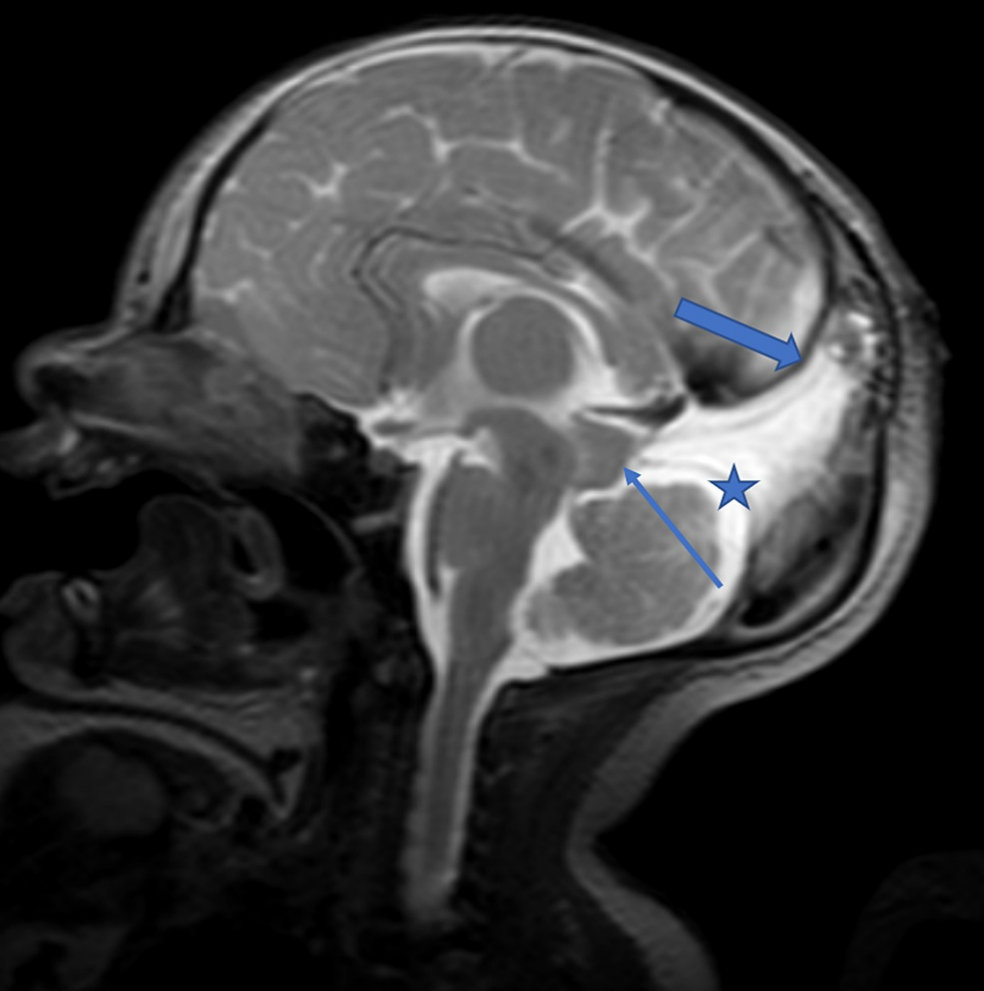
Thick tectum and increased CSF space surrounding the cerebellum T2-weighted sagittal MR image of the brain 15 weeks after the surgery shows the repair of encephalocele and presence of persistent falcine sinus (thick arrow), thick tectum (thin arrow), and excess CSF surrounding the cerebellum (star). CSF: cerebrospinal fluid
